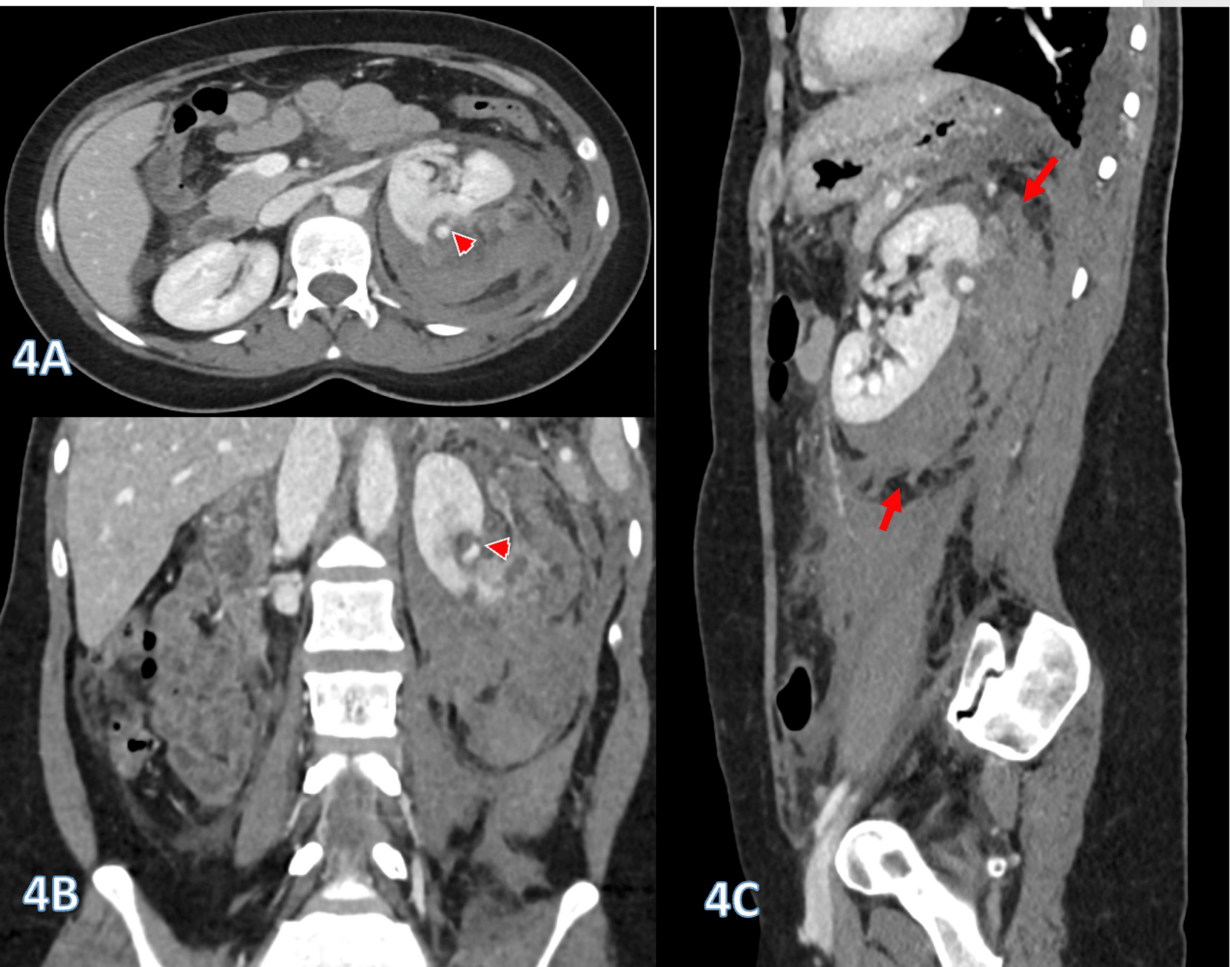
Selected contrast-enhanced CT reconstruction images (4A-4C) demonstrate an extensive perinephric retroperitoneal hematoma (arrows), with an estimated volume of approximately 350 mL and evidence of active bleeding (4B). The bunched-out renal cortex (arrowheads) suggests an underlying cortical lesion, most consistent with AML with a central saccular aneurysm. AML: angiomyolipoma
